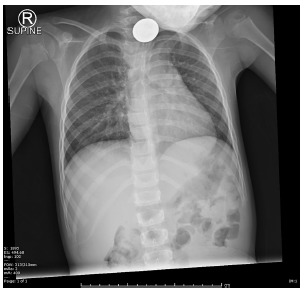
Anteroposterior plain radiograph of the chest, demonstrating disc-shaped foreign body. The en face view suggests but does not prove likely esophageal location.
